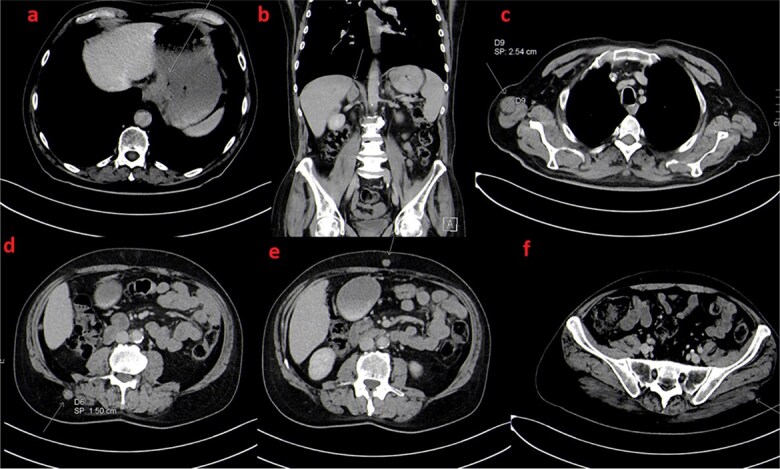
Axial (a, c, d, e and f) and Coronal (b) images from follow up CT scan after 5 months again demonstrate GE junction primary tumor (a). Also, there is progression in the size of many preexisting muscular and subcutaneous deposits (c) in addition to development of new lesions (d, e and f) as well as adrenal metastasis (b).
